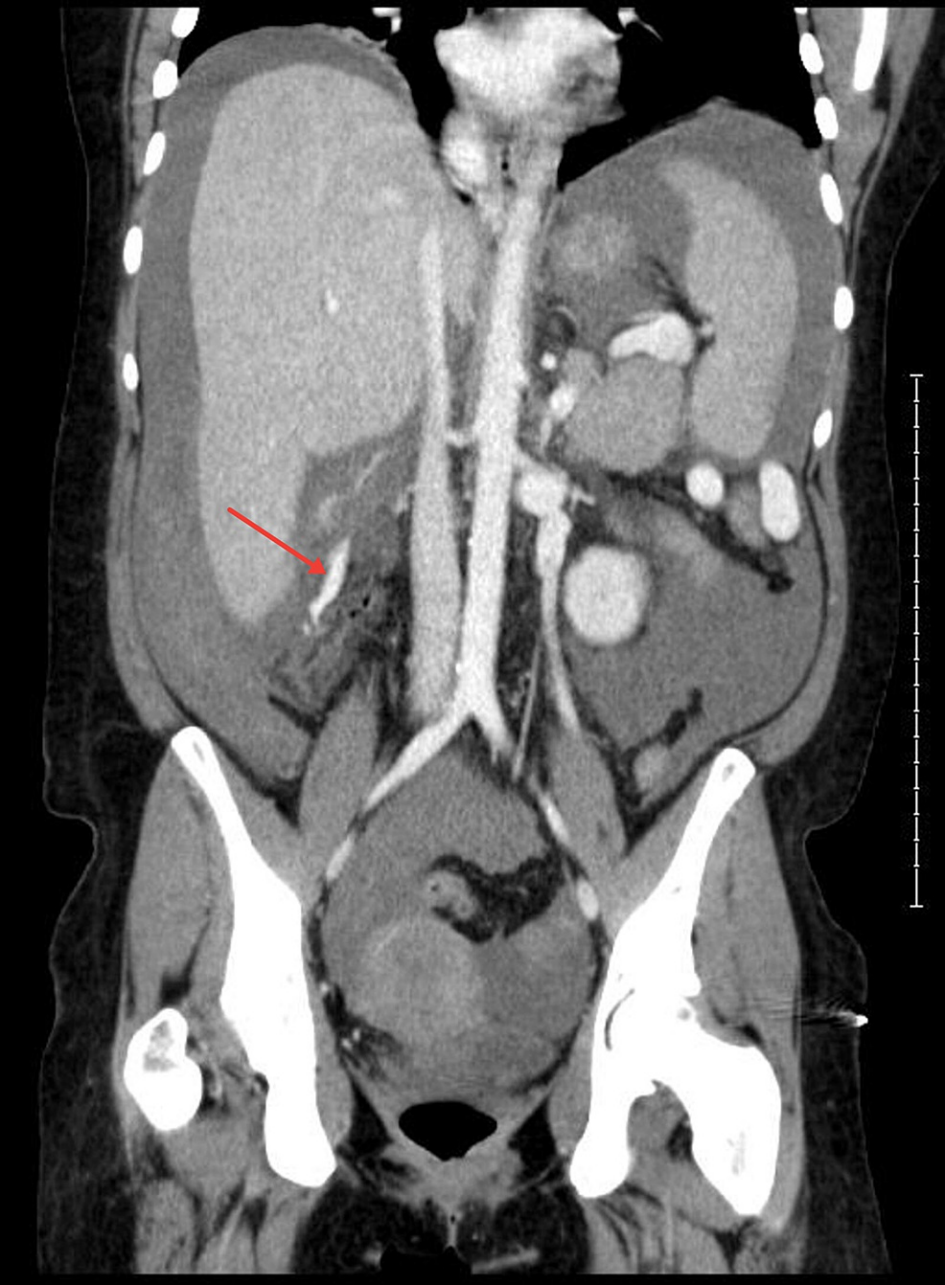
Coronal CT image shows an active extravasation of blood inferior to the right hepatic lobe (arrow). CT, computed tomography.
